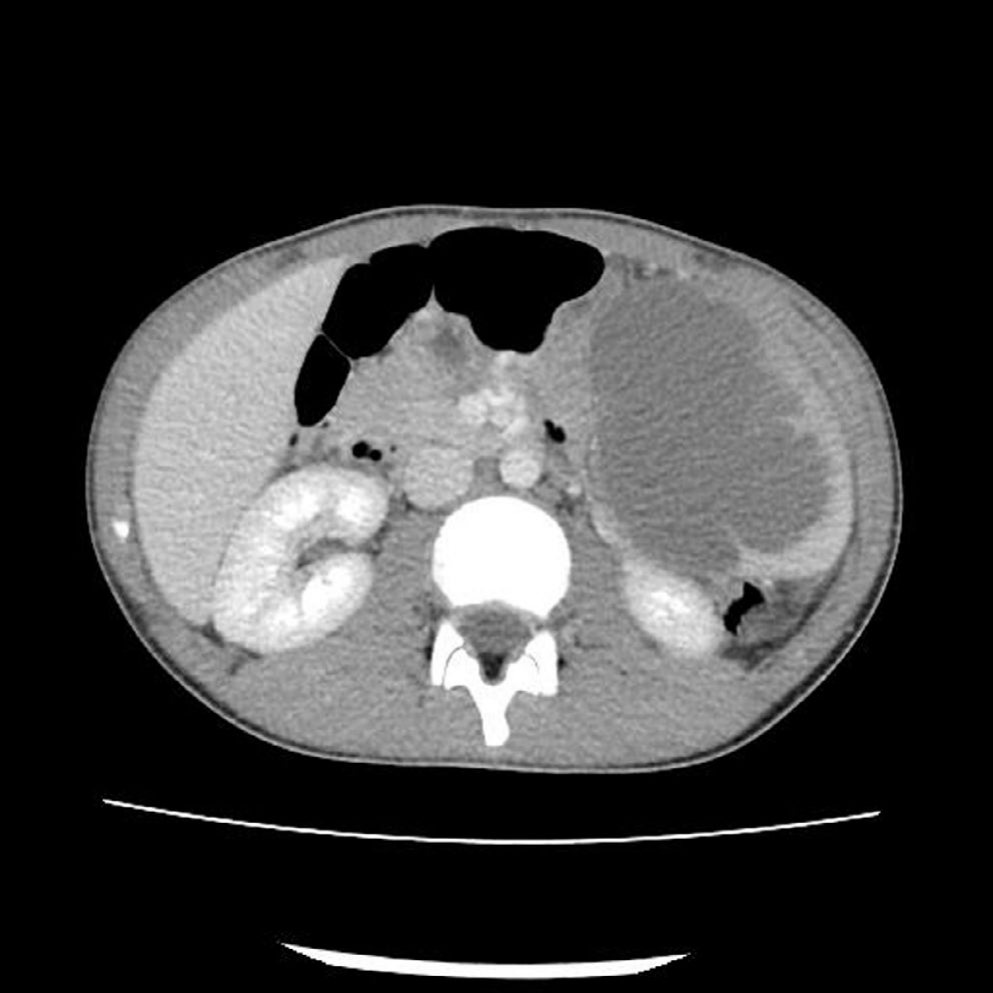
Abdominal CT scan of a 14‐year‐old boy. The spleen contained a large single abscess of 13 cm × 9 cm
